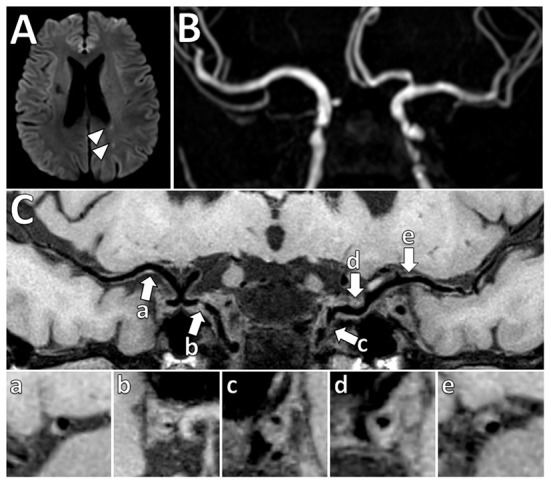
Vessel wall thickening and atherosclerosis on VW-MRI in a symptomatic patient. (A) A patient with left parietal lobe acute ischemic infarcts (arrowheads) underwent intracranial VW-MRI. (B) Time-of-flight MRA and (C) intracranial VW-MRI shows multiple intracranial plaques (arrows) that showed both eccentric (a, b, c, e) and concentric (d) vessel wall thickening. The culprit lesion was thought to be the most stenotic lesion (c) in the left internal carotid artery.
